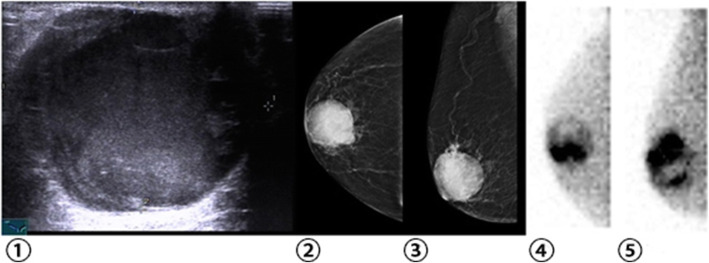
Breast images from an old woman. (①)Ultrasound demonstrated a cystic solid mass in the upper outer region of the right breast, 4.4 cm*3.5 cm; (②③)Mammography demonstrated a high density shadow located in the upper outer region of the right breast, 44 mm*46 mm, BI-RADS 4B; (④⑤)BSGI revealed a focal region of increased radiotracer uptake in the right breast, TNR = 3.59; Pathology identified a 74-mm invasive ductal carcinoma
